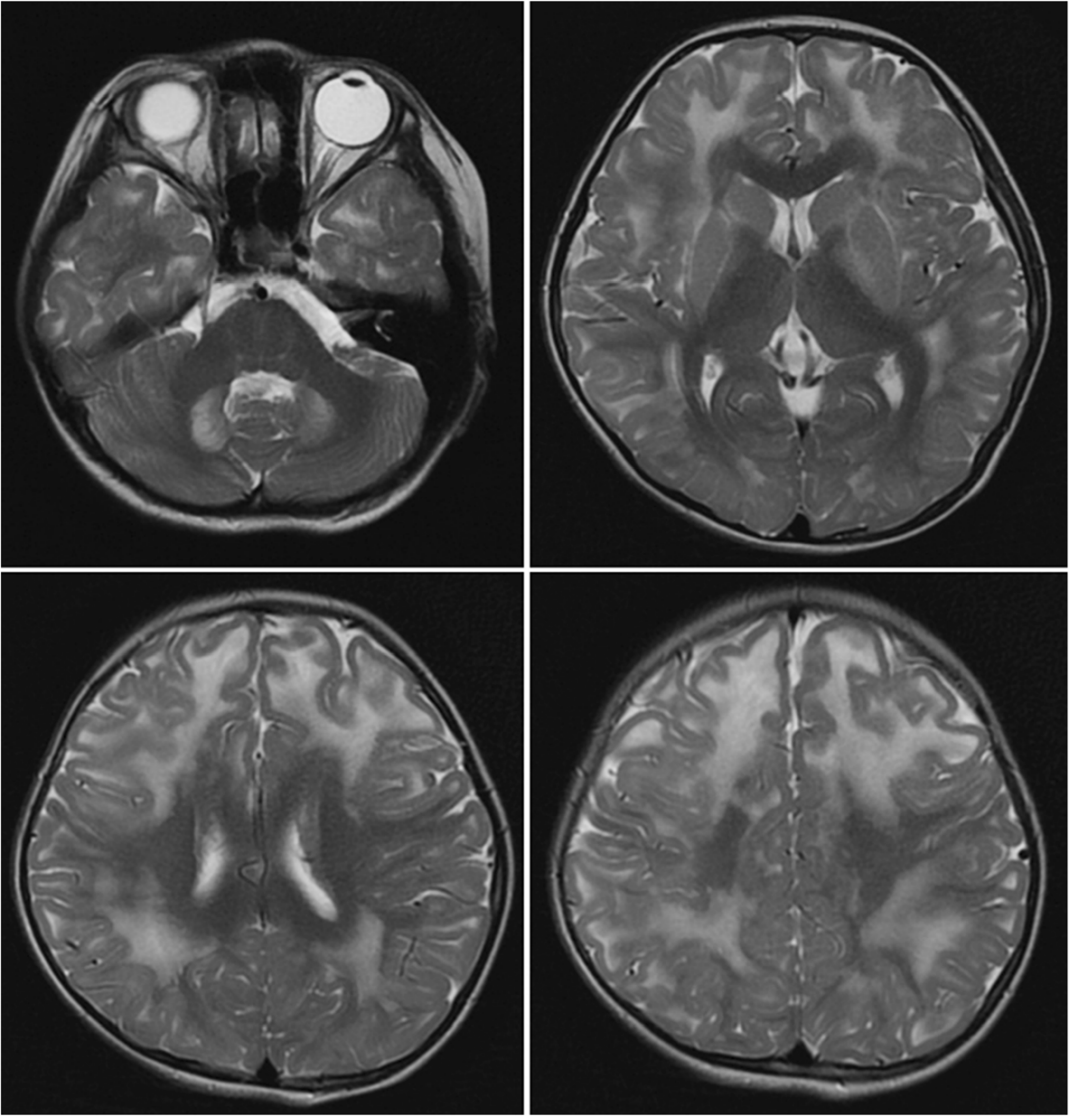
The patient’s brain magnetic resonance image (MRI). Axial T2-weighted sequence of the brain MRI showed symmetrical subcortical white matter hyperintense involving bilateral dentate nucleus, internal capsule, external capsule, and corona radiate
